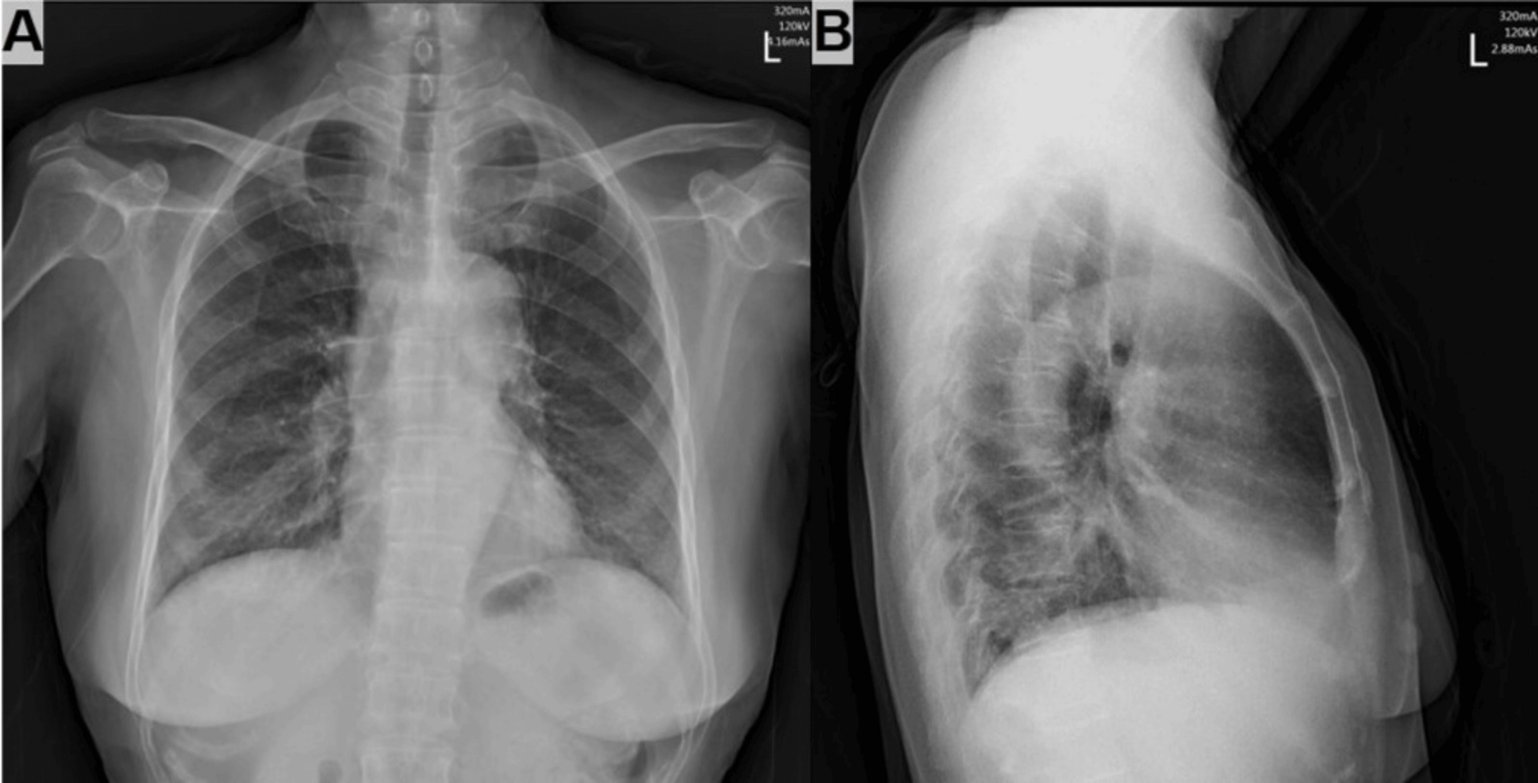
Chest X-ray (posteroanterior view (A) and lateral view (B)) showing mild bilateral interstitial changes.
